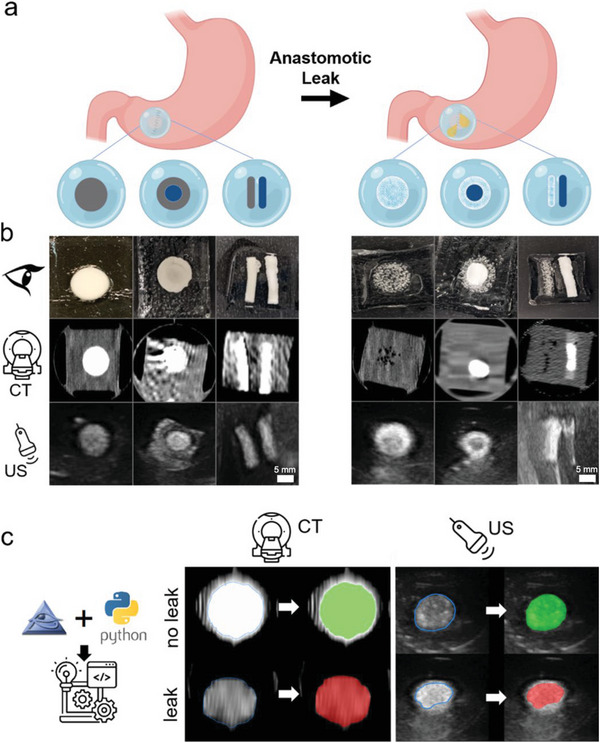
a) (Top) To guarantee easy distinguishability of the dual sensing elements from other anatomical appearances within the human abdomen, reactive geometries are of great benefit. A nonexhaustive list of potential dual sensing element patterns is displayed, made up of reactive and nonreactive components. b) In vitro realization of the above proposed dual sensing element patterns. A nonreacted version (left) is compared to the reacted version (right) visually (top), under computed tomography (CT) (middle), and under ultrasound (US) (bottom). c) Generated CT and ultrasound signal can easily be algorithmically evaluated. By making use of open‐source software, a leak detecting application, capable of classifying the state of the dual sensing element under ultrasound and CT was developed. The application classifies the absolute contrast value of a user selected image region into a leak (red) or no leak (green) category. Representative images from *N* = 3 samples shown.
